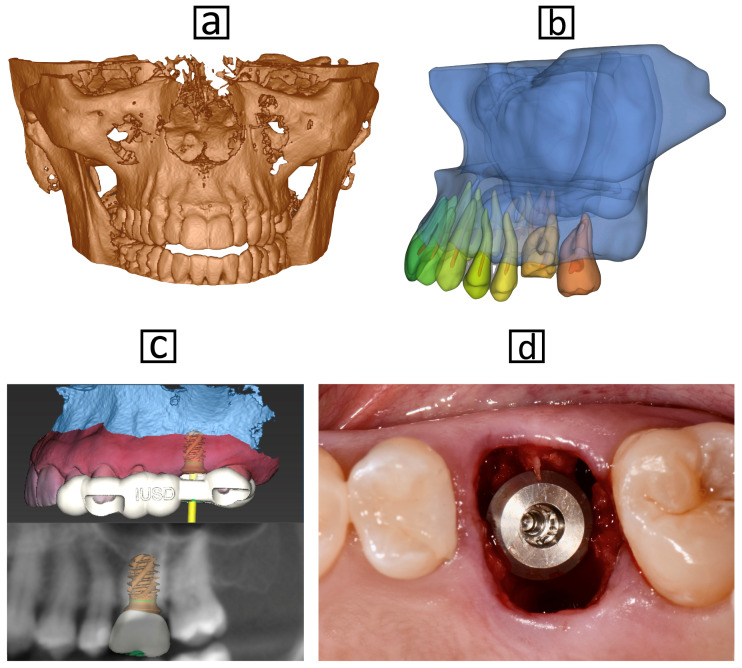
(a) Before segmentation, it is not possible to distinguish anatomical structures in the CBCT images. (b) AI-based automatic segmentation isolating and defining specific anatomical structures within CBCT images efficiently. The segmented dataset can be used for diagnosis, treatment planning, or implant site evaluation, such as extraction and immediate implant placement for the maxillary left first molar in this example. (c) Virtual implant planning using CBCT scans, segmentations, and intraoral scans. (d) Immediate implant placement.
